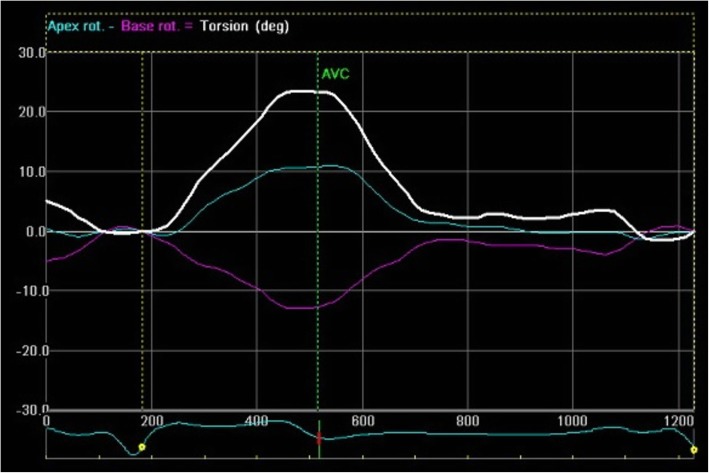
Speckle tracking echocardiography (Echo Pac software) in patients with HCM showing net basal rotation (purple color) in counter-clockwise direction and net apical rotation (green color) in clockwise direction. Net torsion is displayed in white color
